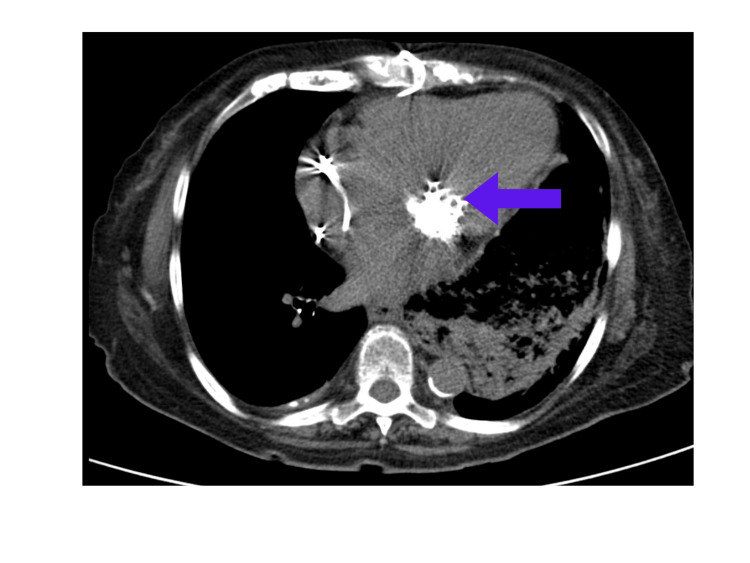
Computed tomography chest three months after mitral valve surgery. The blue arrow shows the replaced mitral valve.
